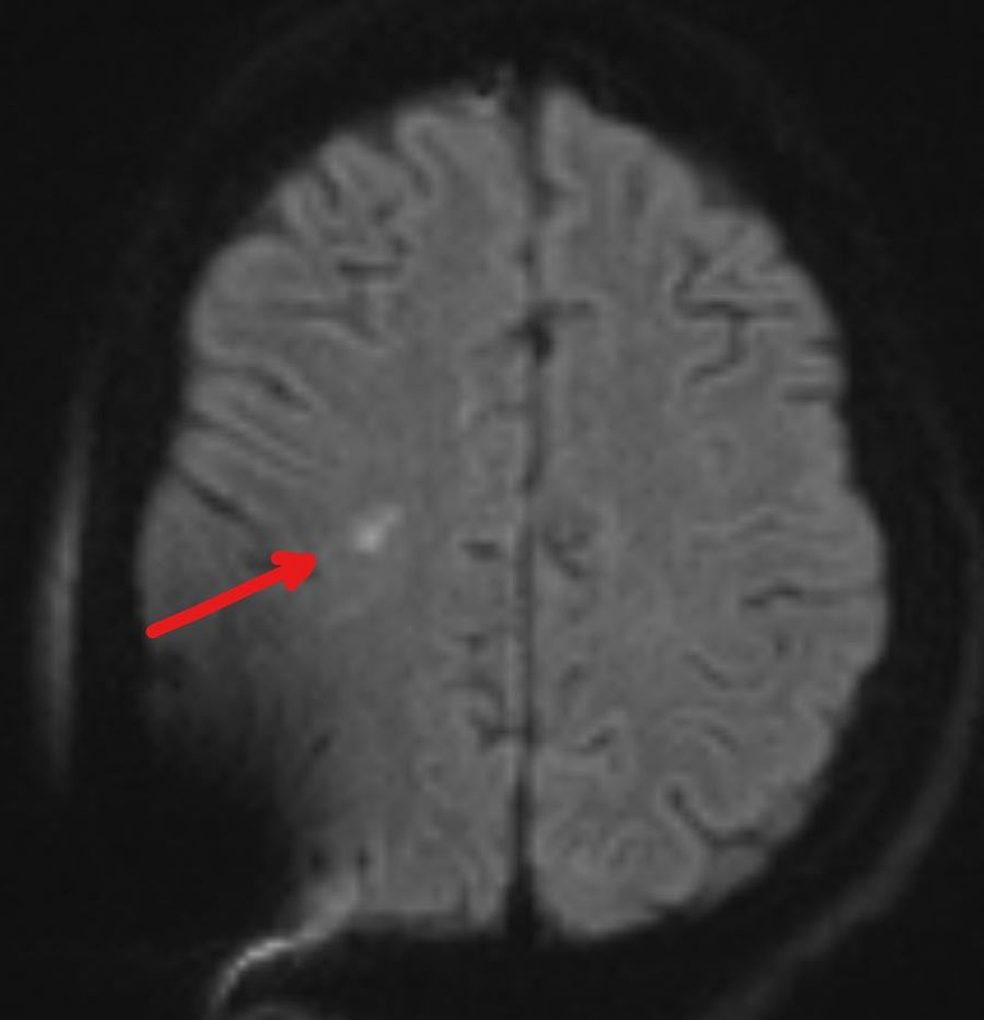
DWI of head MRI, Day 6. DWI: diffusion-weighted imaging.
